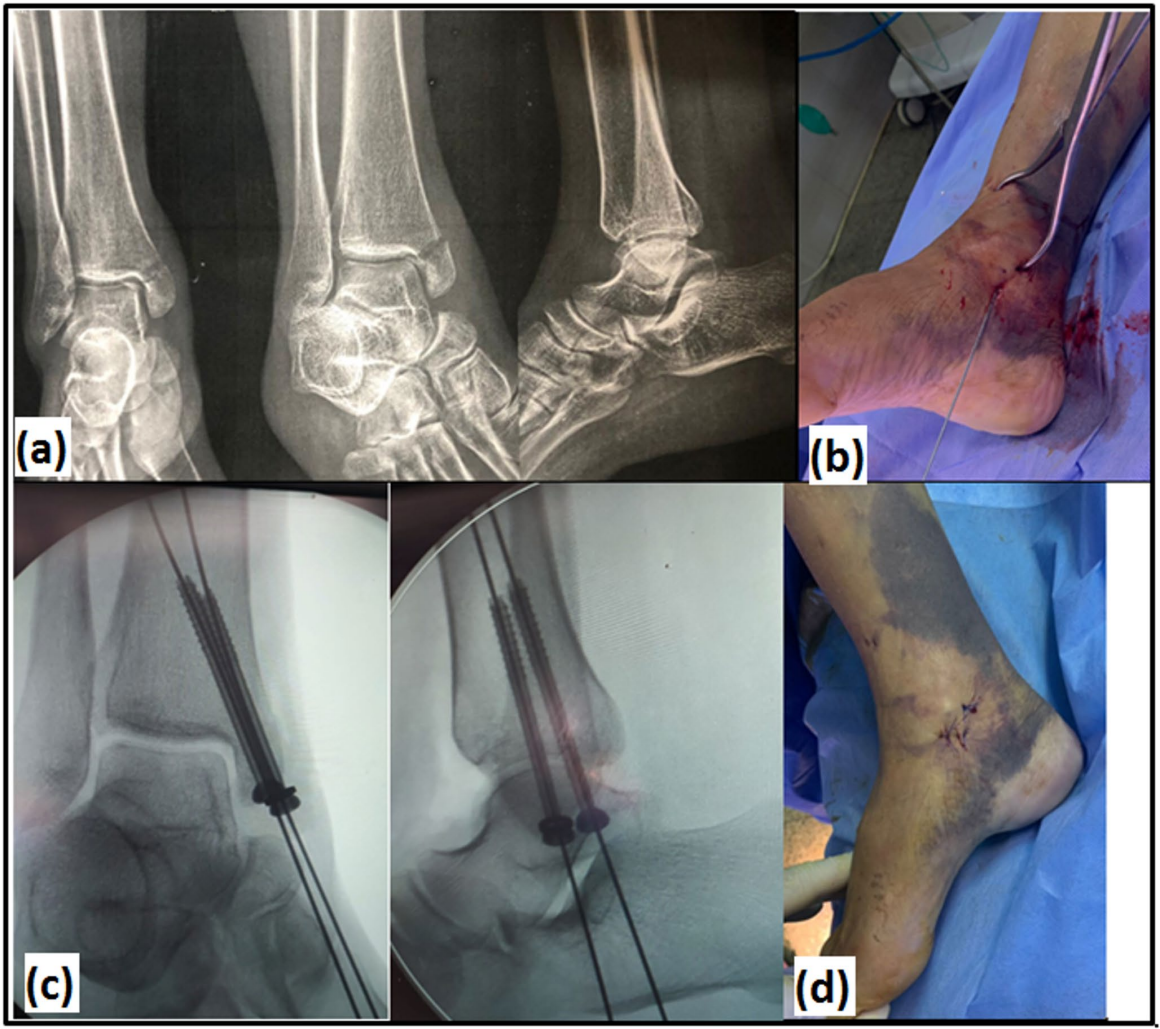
Surgical technique of CRPF: a pre-operative AP and lateral X-ray views of a patient with isolated MM fracture. Intra- operative photographs demonstrating; b holding the reduction by pointed reduction forceps applied from tip of MM perpendicular to fracture line, and then guide wires for cannulated screws were inserted one after another, c C-arm fluoroscopy showing cannulated screws insertion over guide wires d simple skin stitches for stab incisions
